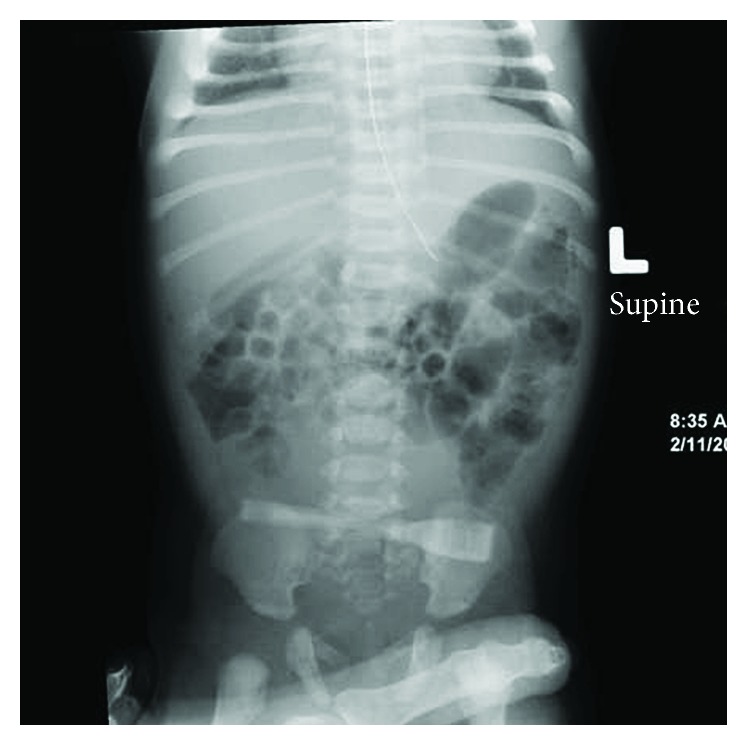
Abdominal radiograph showing an air-filled stomach, duodenum, and jejunum.
